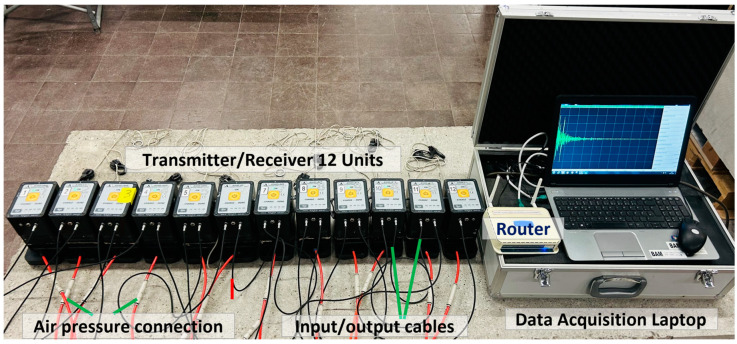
Large Aperture Ultrasound System (LAUS), 12 units placed equidistantly.
